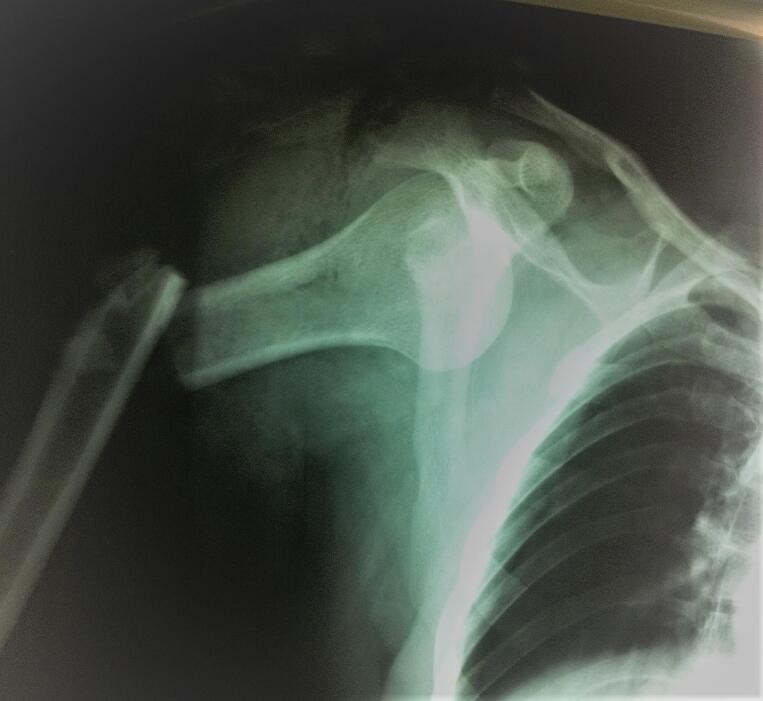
X-ray showing anterior dislocation of the right shoulder with ipsilateral shaft humeral fracture.
